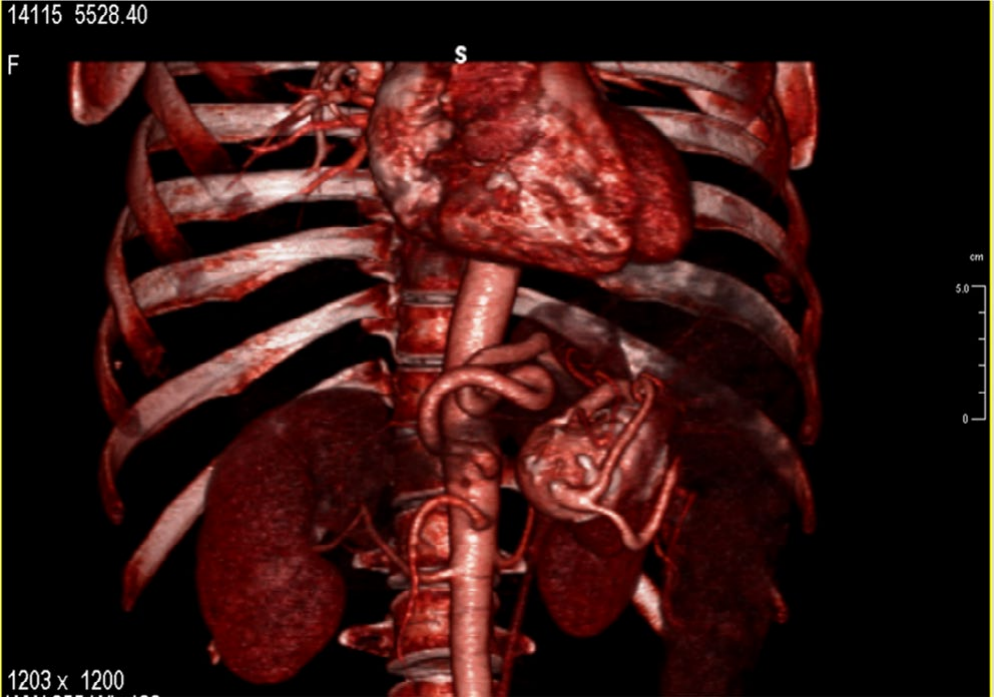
Three‐dimensional reconstruction of abdominal computed tomography scan.
